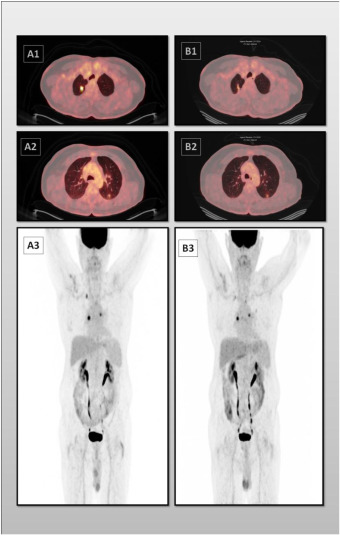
[Supplementary Figure 1: Axial fused PET‐CT and whole‐body maximum intensity projection (MIP) images before (A1–A3) and after (B1–B3) radiofrequency ablation (RFA).(A1, A2) Axial fused PET‐CT images demonstrate an 11 × 11 mm FDG‐avid nodule in the apicoposterior segment of the right upper lobe (SUVmax = 7.3, blood pool = 3.9) and a 9‐mm FDG‐avid nodule in the apical segment of the left lower lobe (SUVmax = 2.1, blood pool = 2.2). (A3) Whole‐body MIP image shows bilateral pulmonary FDG uptake without additional abnormal findings. (B1–B3) Post‐treatment PET‐CT images following RFA demonstrate a marked reduction in metabolic activity of the treated lesion, with no evidence of new abnormal FDG uptake elsewhere in the body.]
